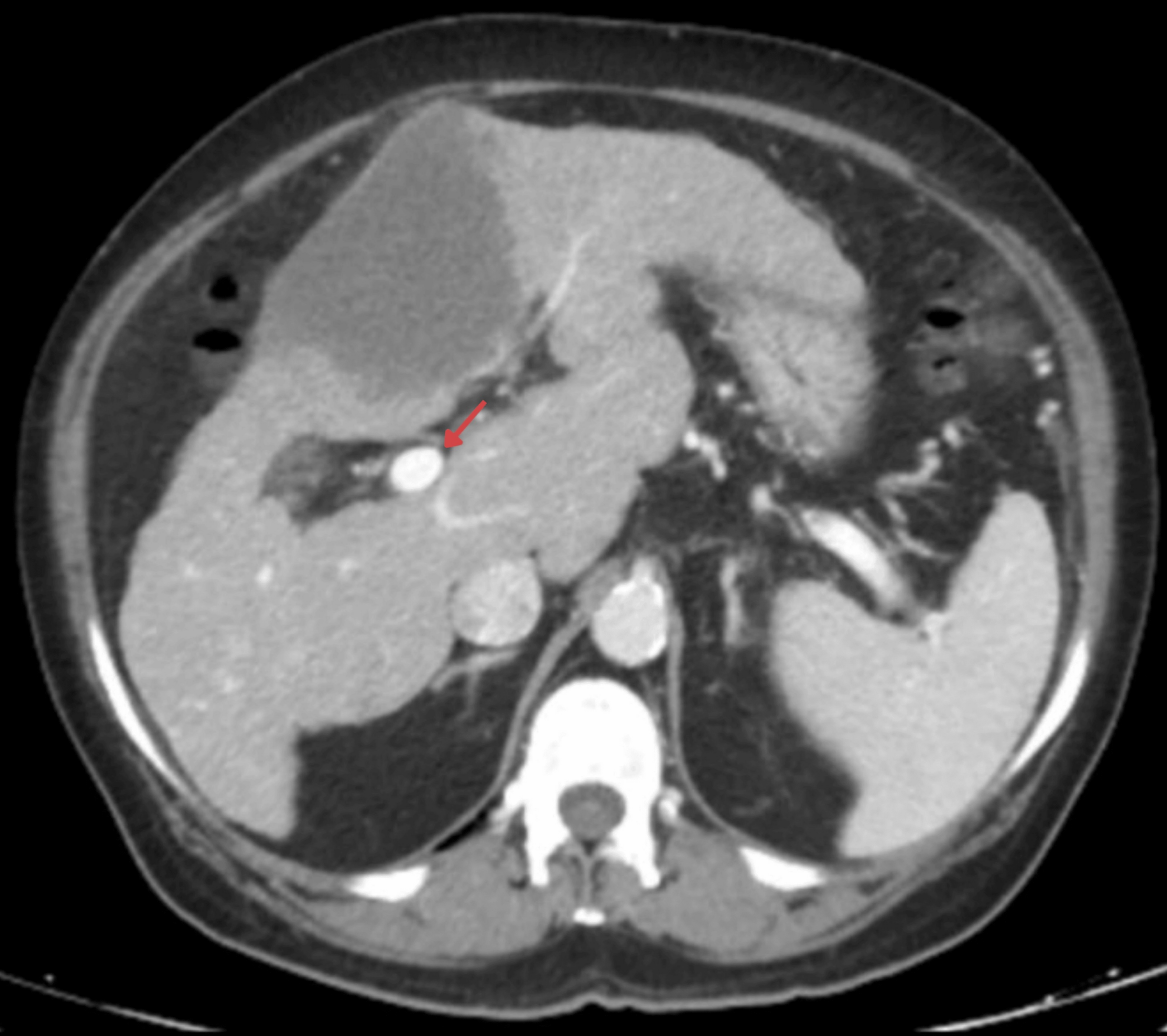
Axial contrast-enhanced CT of the abdomen (portal venous phase) demonstrating opacification of the portal vein (arrow), indicating absence of macrovascular invasion, a feature commonly seen in hepatocellular carcinoma and cholangiocarcinoma, thereby supporting the exclusion of these differentials.
